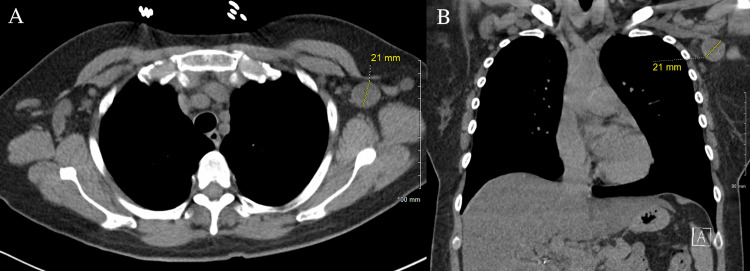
(A) Axial CT chest demonstrating enlarged axillary left lymph node with central low-density concerning for necrosis. (B) Coronal CT chest demonstrating enlarged axillary left lymph node.
